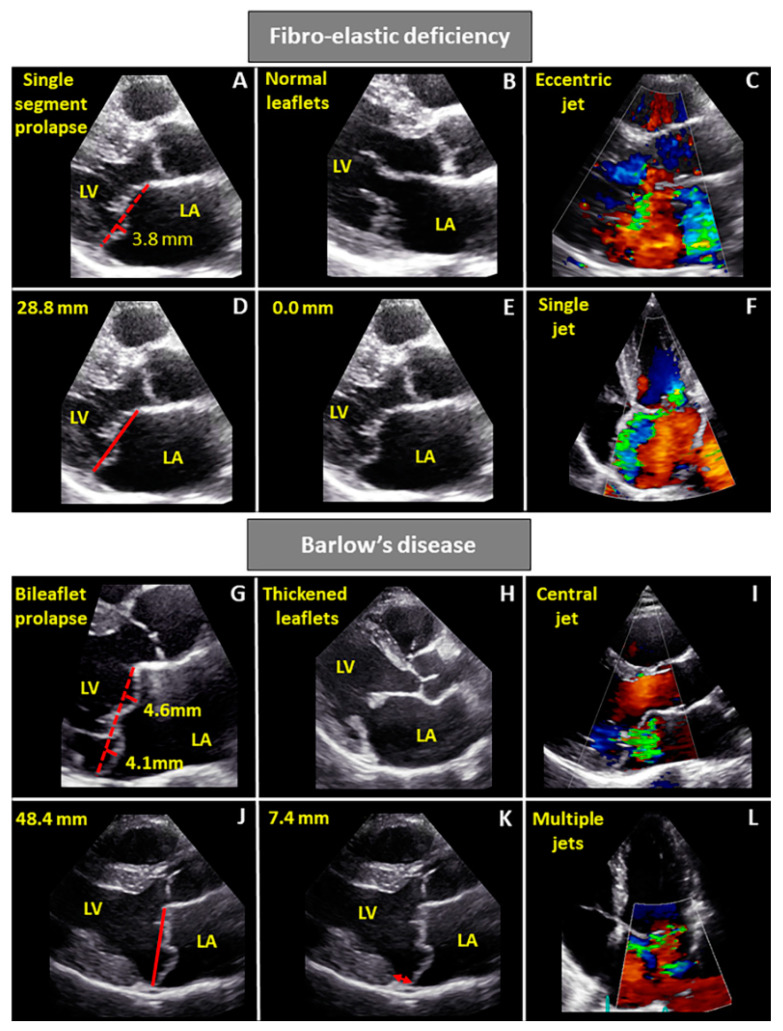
Transthoracic echocardiography: Differences between fibroelastic deficiency and Barlow’s disease. Transthoracic echocardiography images (parasternal long-axis view (A–E,G–K) and four-chamber view (F,L)) presenting the differences between FED and Barlow’s disease. Panels A and G displays the difference in prolapsing segment, including the prolapse height. Patients with FED have a single segment prolapse compared to the bileaflet prolapse of Barlow’s disease patients. As displayed in panels B and H, patients with Barlow’s disease have thick leaflets compared to the thin leaflets in FED. Panels D and J demonstrate that the mitral valve annulus diameter is larger in Barlow’s disease compared to FED, where the annulus is within the normal range. Patients with Barlow’s disease often have MAD, as presented in panel K with the red arrow, whereas this is rare in patients with FED (panel E showed no signs of MAD). Panels C, F, I and L show = mitral regurgitation in both patients, with patients with Barlow’s disease more often having multiple jets compared to the single jet in FED patients. FED = fibroelastic deficiency, LA = left atrium, LV = left ventricle and MAD = mitral annular disjunction.
